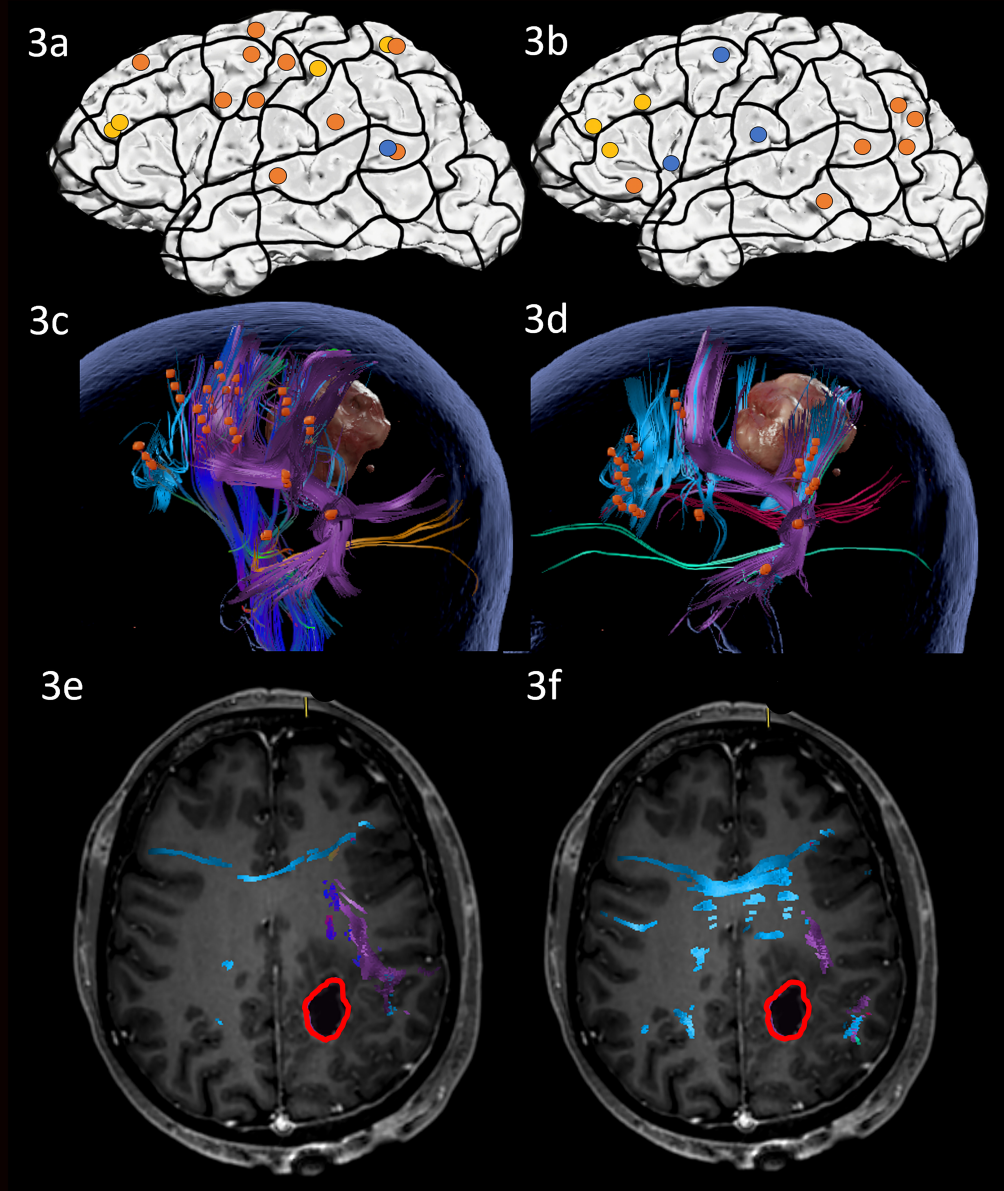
Case 3. Left panels show ON results, right panels AN results. Panels (A, B) nTMS stimulation sites that induced errors; no response and hesitations in orange, semantic errors in yellow and performance errors in blue. Panels (C, D) Fiber tracking results including the lesion, nTMS cortical endpoints in orange and color-coded tracts with CNT in direction-coded, here dark blue, AF in purple, SLF in pink, IFOF in turquoise, ILF in yellow, and CF in light blue. Panels (E, F) Overlap of tracking and postoperative T1-weighted imaging after elastic with the resection cavity marked in red.
